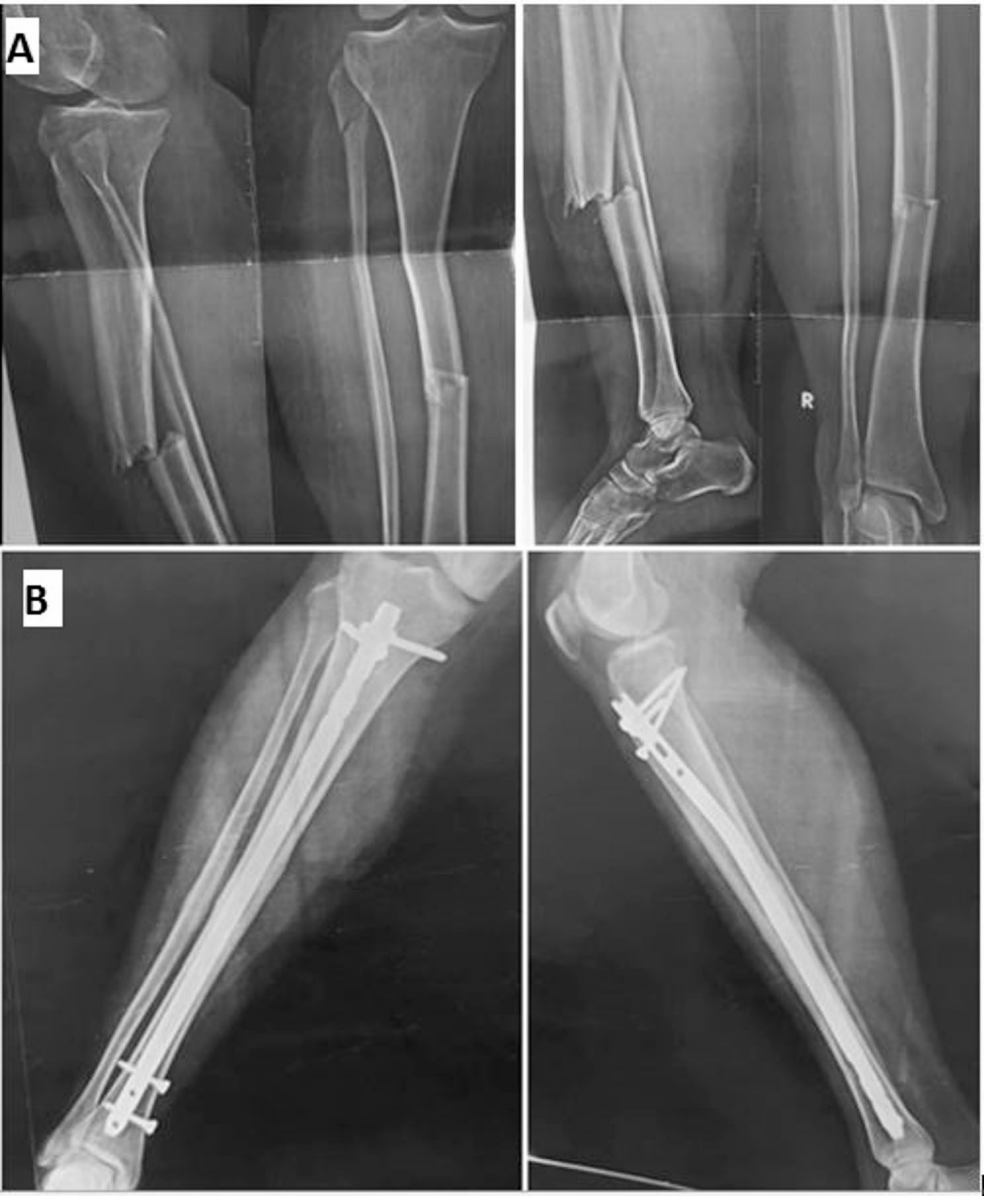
Surgical technique of standard static ILN: A Pre-operative AP and lateral right leg radiographs of a 30 years old female patient showing middle third tibial shaft fracture, AO type 42-A3. B Follow up radiographs at the 4th month showing complete bony union
